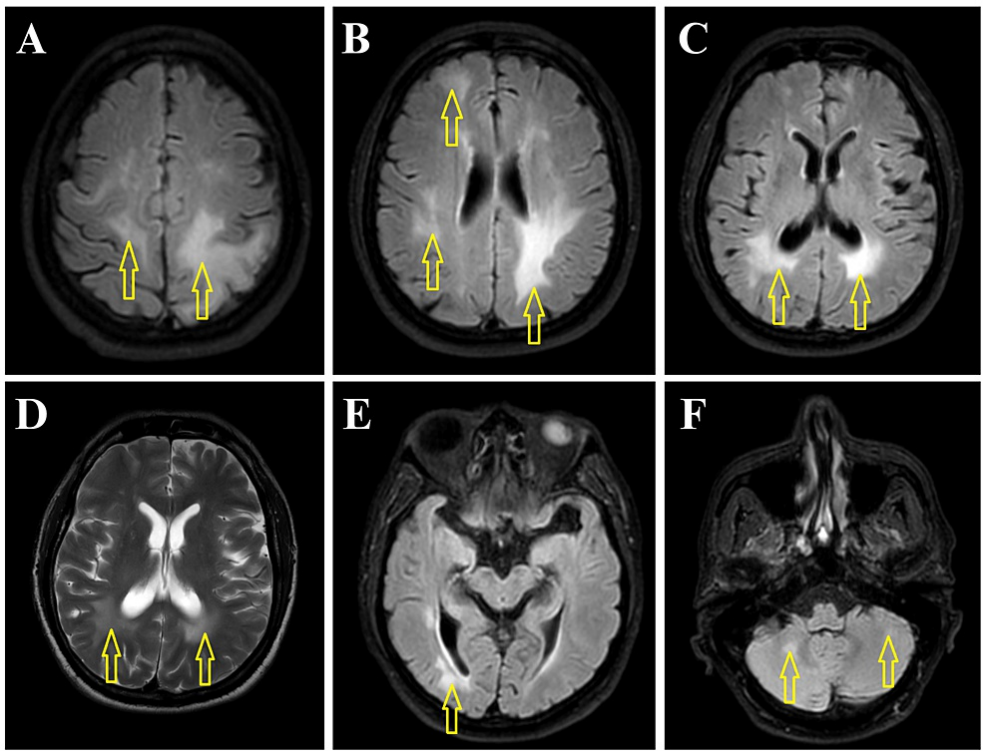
Axial FLAIR (1A, 1B, 1C, 1E, 1F) and T2W (1D) MRIs of the brain at different levels show bilateral asymmetrical patchy hyperintense lesions (arrows) in the periventricular and subcortical white matter of both cerebral hemispheres (1A to 1E) and the white matter of both cerebellar hemispheres (1F). FLAIR: fluid-attenuated inversion recovery; T2W: T2-weighted; MRI: magnetic resonance imaging
